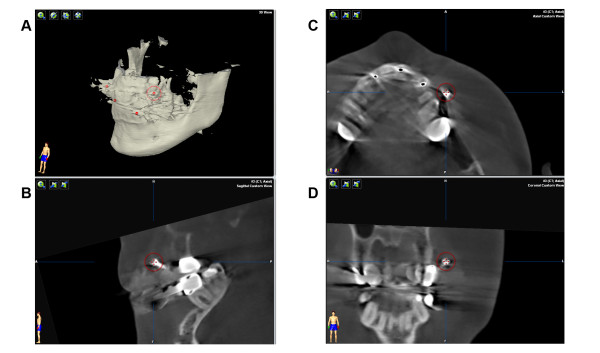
3D image for tumor diagnostic. To enable Computer-assisted surgery, tumor diagnostic 3D imaging has to be complemented with a marked 3D data set. The authors recommend a cone beam CT scan subvolume with included navigation splint.
